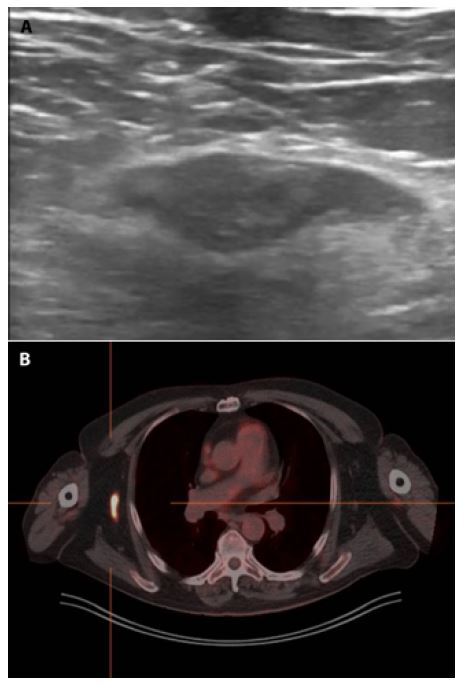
Lymph node metastasis of cutaneous squamous cell carcinoma on the right arm. (A) Regional ultrasound shows a 17 mm hypoecoic structure also identified in the PET-CT scan. (B) The patient undewent right axillary lymph node dissection.
